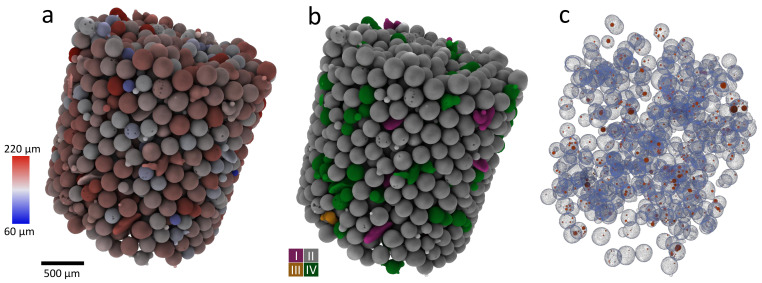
Micrometre-size glass bead quantification with X-ray CT. (a) Glass beads coloured according to equivalent diameter from blue (60 μm) to red (220 μm). (b) The same glass beads coloured according to Zingg’s [66] shape classification (discs (I): purple, spheres (II) grey, blades (III): orange, rods (IV): green). (c) Only beads with internal pores shown (beads in blue, pores in red).
